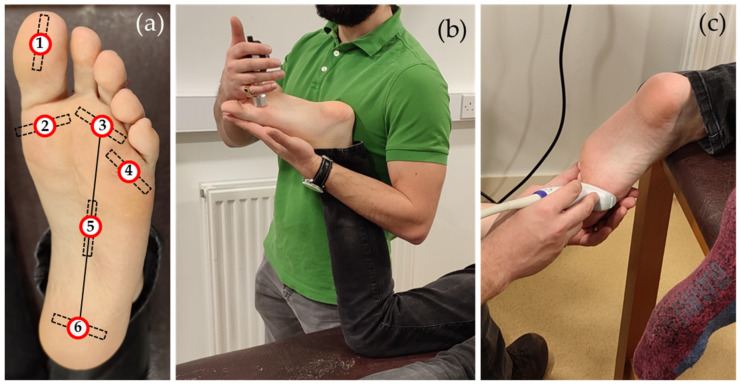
(a) The measurement sites and the orientation of the ultrasound probe during SW imaging at the hallux (1), 1st metatarsal head (MetHead) (2), 3rd MetHead (3), 5th MetHead (4), midfoot (5) and rearfoot (6). The testing set-up of SH testing (b) and SW elastography imaging (c).
